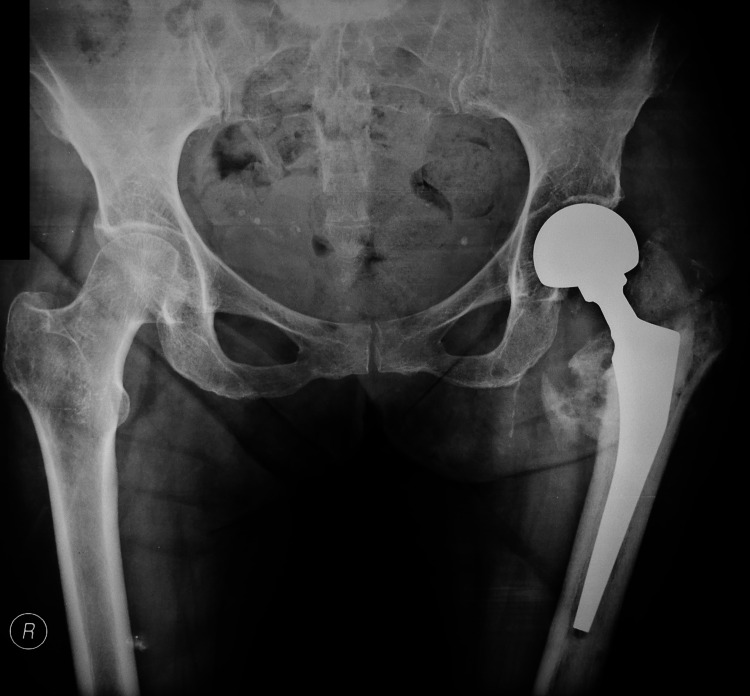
Anteroposterior pelvis x-ray, one month postoperatively.
